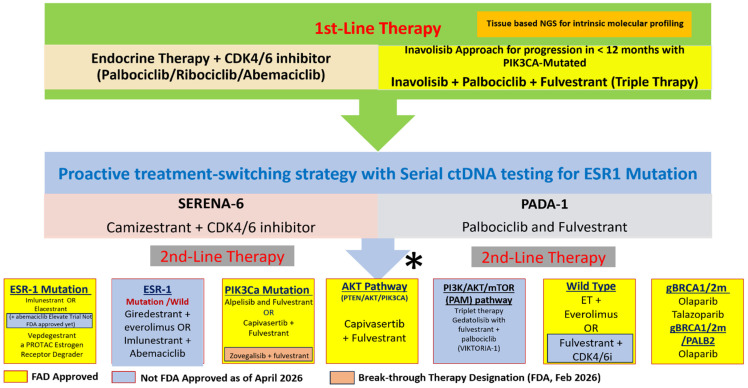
Proposed biomarker-directed treatment algorithm for HR+/HER2− mBC following CDK4/6 inhibitor progression. First-line therapy consists of endocrine therapy plus a CDK4/6 inhibitor. Comprehensive molecular profiling using tissue-based NGS and/or circulating tumor DNA (ctDNA) is recommended at baseline and at progression to guide treatment selection according to dominant molecular alterations, including ESR1, PIK3CA, AKT/PTEN/PIK3CA pathway alterations, PI3K/AKT/mTOR pathway activation, gBRCA1/2/PALB2, and wild-type disease. Yellow boxes indicate FDA-approved treatment options, purple boxes indicate investigational or not-yet-FDA-approved approaches as of April 2026, and the orange box indicates agents or combinations with FDA breakthrough therapy designation. In patients with PIK3CA-mutated tumors and early progression, including recurrence while on or within 12 months of completing adjuvant endocrine therapy, inavolisib-based triplet therapy represents an evidence-supported strategy. The middle section highlights proactive serial ctDNA monitoring for emergent ESR1 mutations, enabling early treatment switching before radiographic progression, as evaluated in SERENA-6 with camizestrant plus a CDK4/6 inhibitor and PADA-1 with fulvestrant plus palbociclib. Investigational strategies are indicated where applicable.  In patients with visceral crisis, rapid symptomatic progression, endocrine-refractory disease, or exhaustion of biomarker-directed endocrine-based options, transition to single-agent chemotherapy or ADC therapy should be considered, with trastuzumab deruxtecan favored for HER2-low/HER2-ultralow disease and sacituzumab govitecan considered in later-line HER2-negative disease*.
